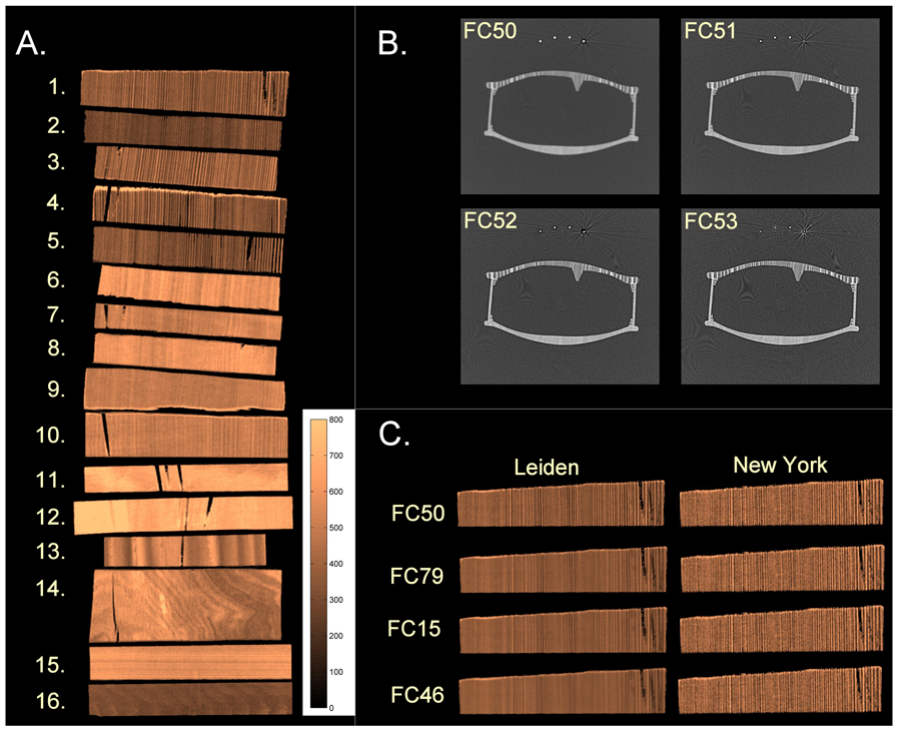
Protocol optimization. a. Calibration wood-set composed of different wood sample pieces; #'s 1–5,16, picea abies, #'s 6–10,15, acer pseudoplatanus, #11 fagus grandifolia, #12 acer glabrum, #13 populus albus, and # 14 juglans nigra. b. Comparing differences in metal artifacts from the metal strings in a CT scan of a violin; c. Images of the first calibration wood piece, obtained from the four candidate filters.
